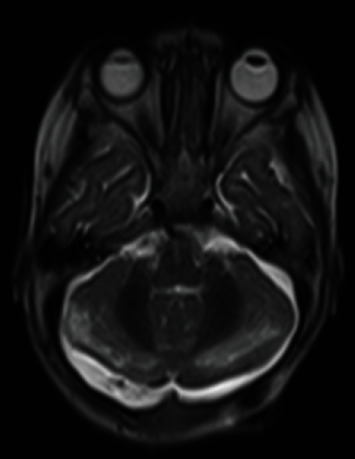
MRI evaluation 3 weeks after initial presentation. Axial T2-weighted image through the cerebellum three weeks after presentation shows volume loss and bright signal in the cerebellar hemispheres consistent with encephalomalacia in the regions of previous cerebellar injury and diffusion restriction.
